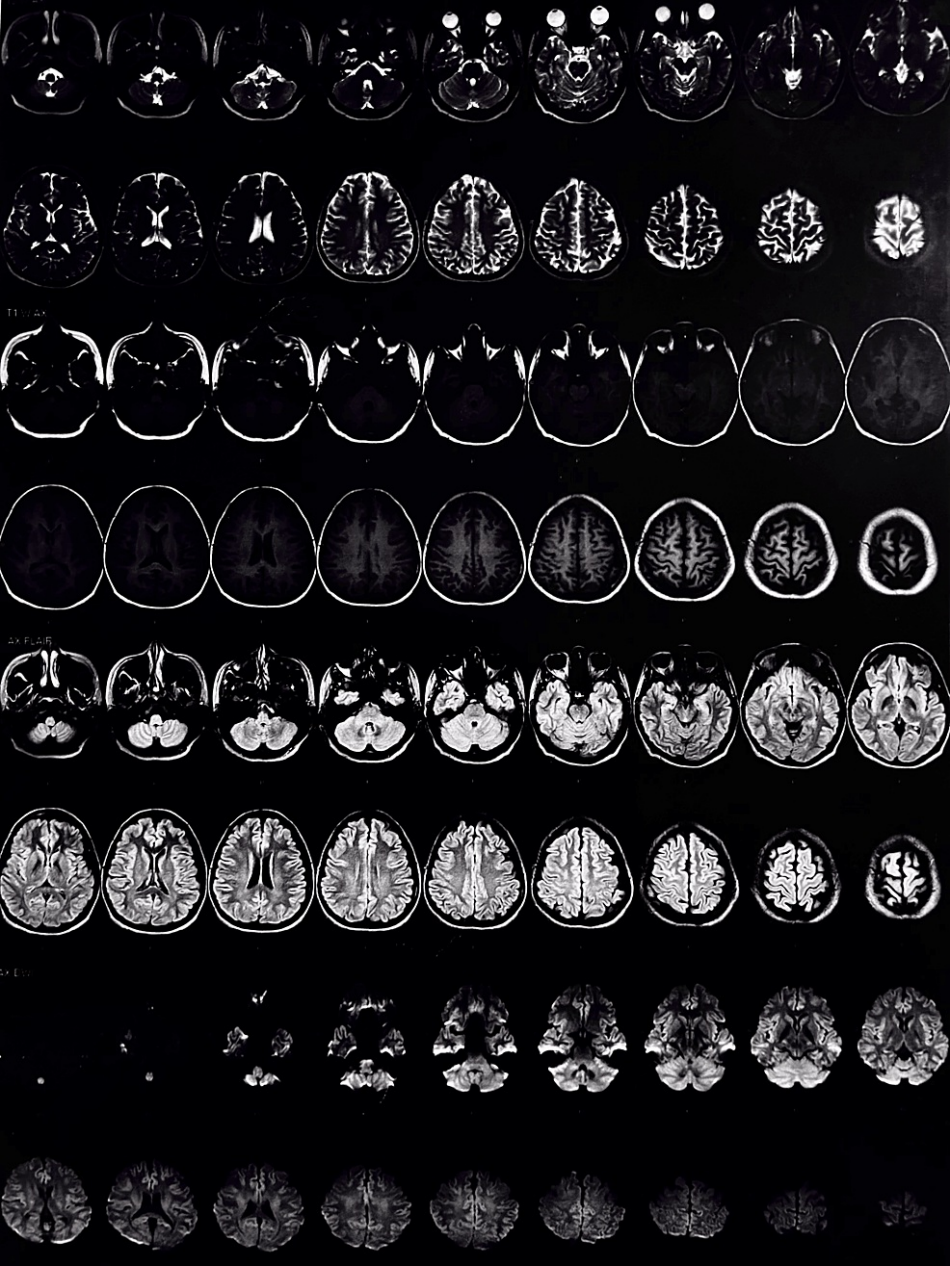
MRI scan of the patient showing no deformities and normal cerebral activity
